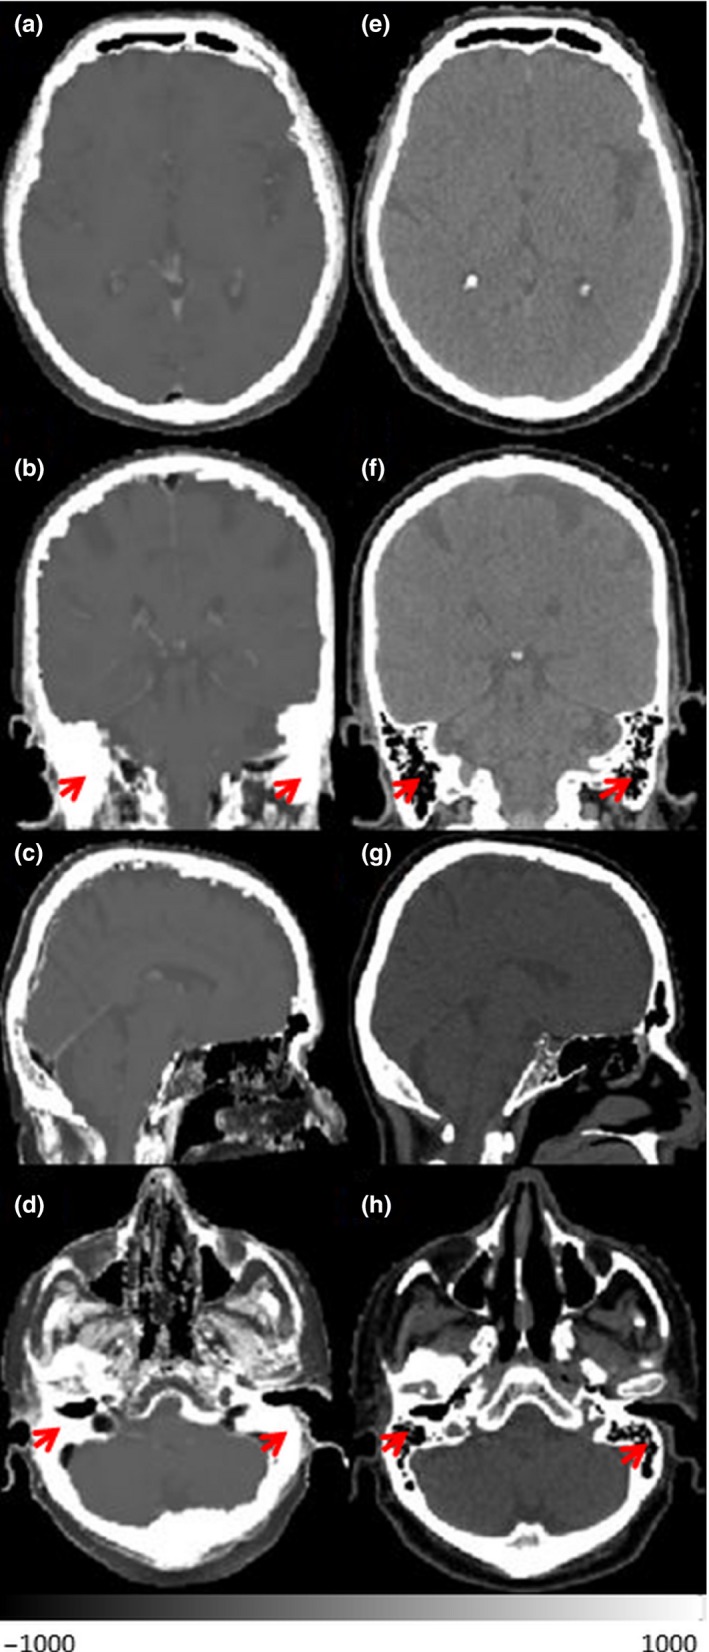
Synthetic‐CT (a–d) and corresponded CT (e–h) in three views and different axial level of one patient. Red arrows indicate mastoid sinuses
